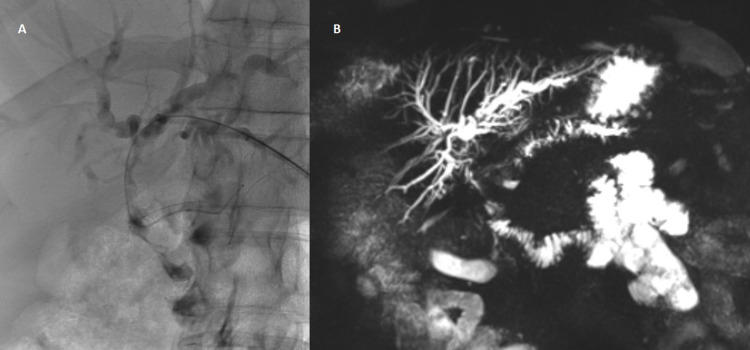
Cholangiography (A) and cholangio-MRI (B) showing dilatation of intrahepatic bile ducts.
